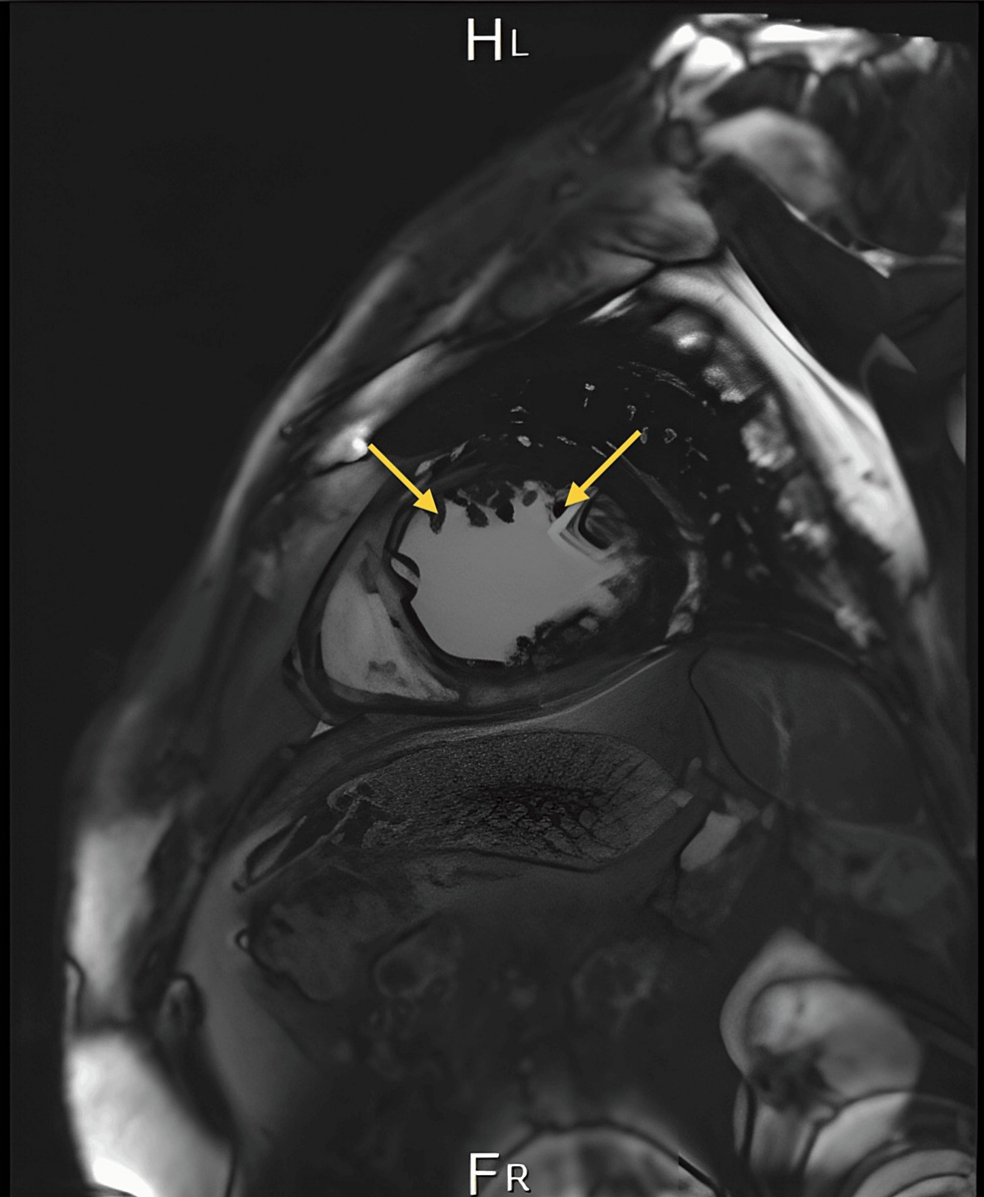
MRI demonstrated typical morphology of left ventricular non-compaction cardiomyopathy, prominent in the left ventricle endocardium with more than 3 villi below the papillary muscle (view 1).
